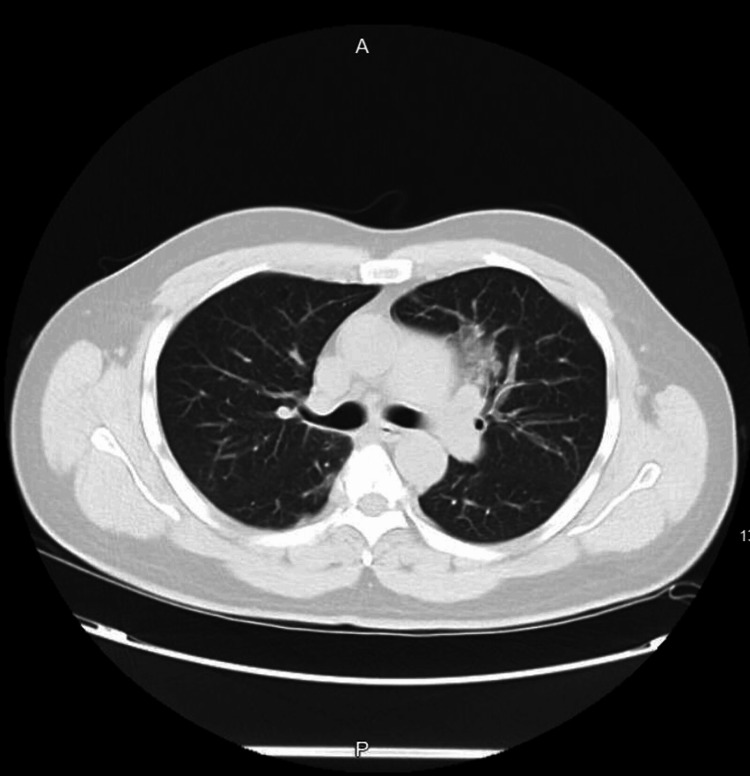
Chest CT showing ground-glass opacity in the anterior left upper lobe
